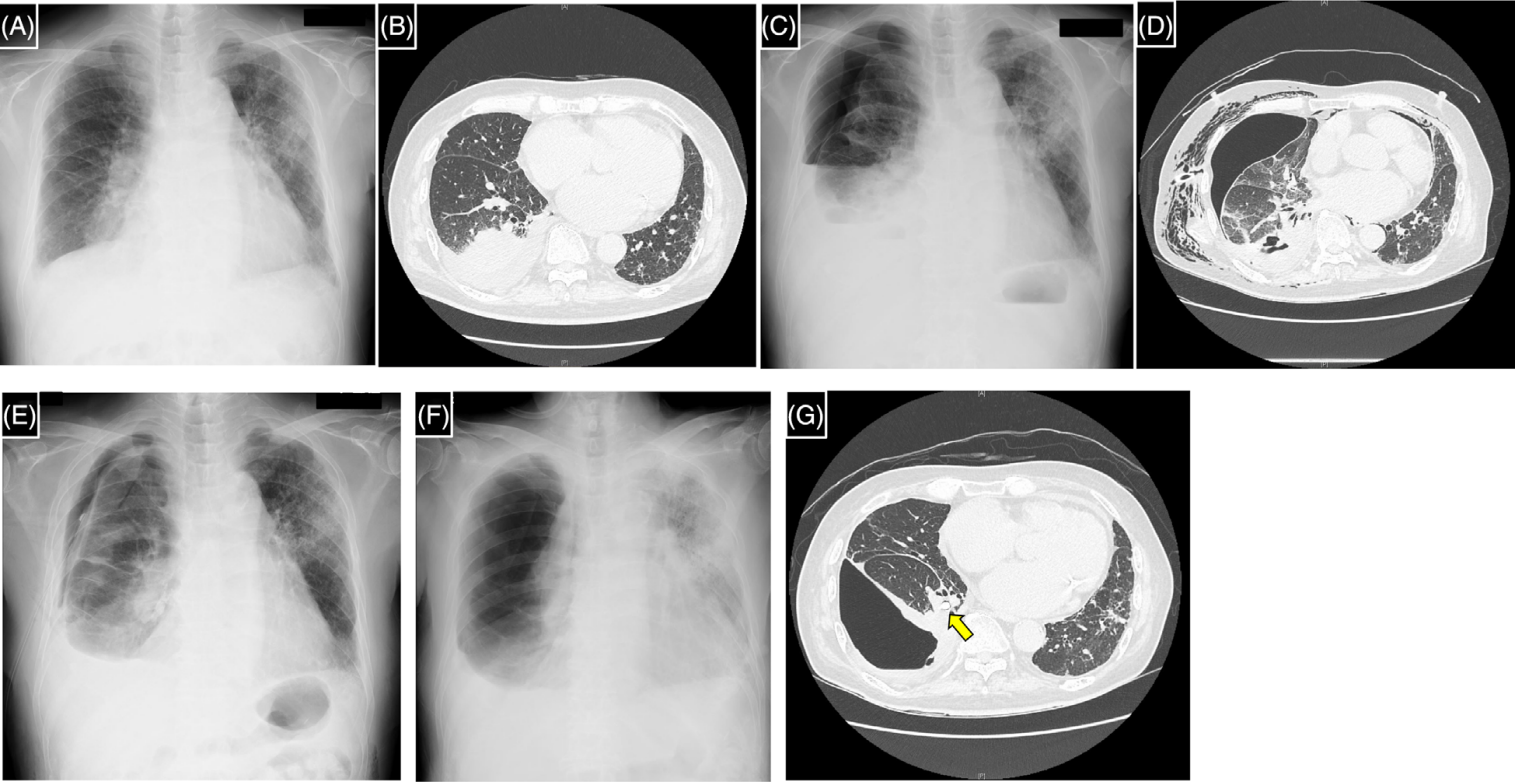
Chest radiograph and computed tomography (CT) of the patient performed at the time of admission (A, B). Chest radiograph performed when the pneumothorax occurred (C). CT examination, performed after pneumothorax was observed on chest radiograph, showing the dilated right B9/10 which appeared to connect with the thoracic cavity (D). Chest radiograph performed after the bronchial occlusion was treated using a combination of Endobronchial Watanabe Spigot and N‐butyl‐2‐cyanoacrylate (E). Chest radiograph and CT performed when right‐sided pneumothorax re‐emerged and left‐sided ground‐grass opacity worsened (F, G). The yellow arrow shows the remaining EWS in the right B9 (G).
